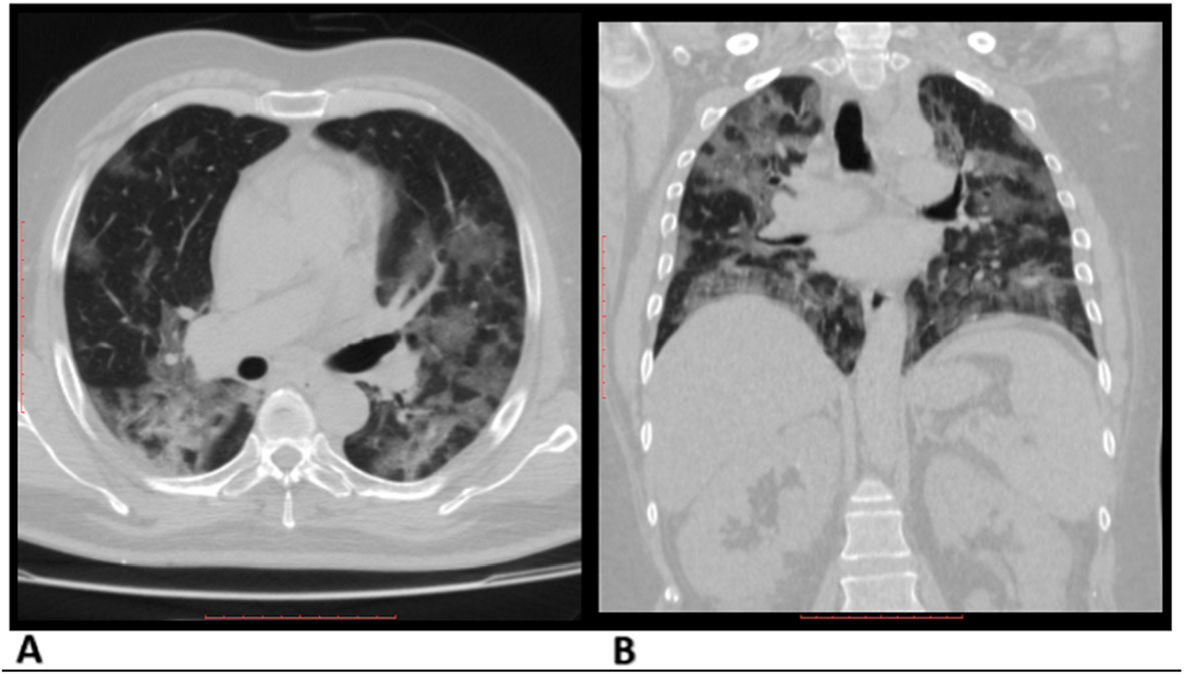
CT typical findings of COVID-19 affection. A 48-year-old man with multiple ground-glass lesions and a crazy-paving pattern. Observers agreed on COVID-RADS score 3 and CO-RADS score 5. The preference of COVID-RADS: CO-RADS was (2:1). RT-PCR result was positive
